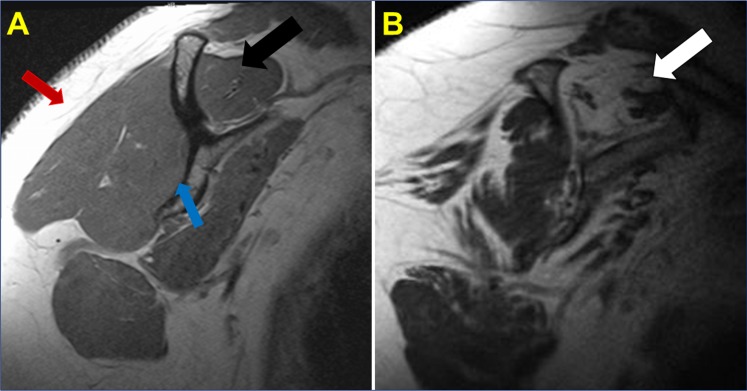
(A) T1 Sagittal magnetic resonance imaging (MRI) image of healthy rotator cuff musculature. Normal muscle appears dark on a T1 sequence, in contrast to the subcutaneous fat that appears light (white arrow). The black arrow denotes the healthy supraspinatus muscle belly without atrophy or fatty infiltration. The blue arrow points towards the body of the scapula. (B) Sagittal oblique T1 image of the shoulder in a patient with a chronic, massive rotator cuff tear. In contrast, T1 Sagittal MRI image of the shoulder demonstrates significant fatty infiltration and atrophy of the supraspinatus in the setting of a chronic, massive rotator cuff tear. Note the fatty infiltration of the supraspinatus tendon (white arrow) where the muscle has a similar white radiographic appearance as the subcutaneous fatty tissue (red arrow).
